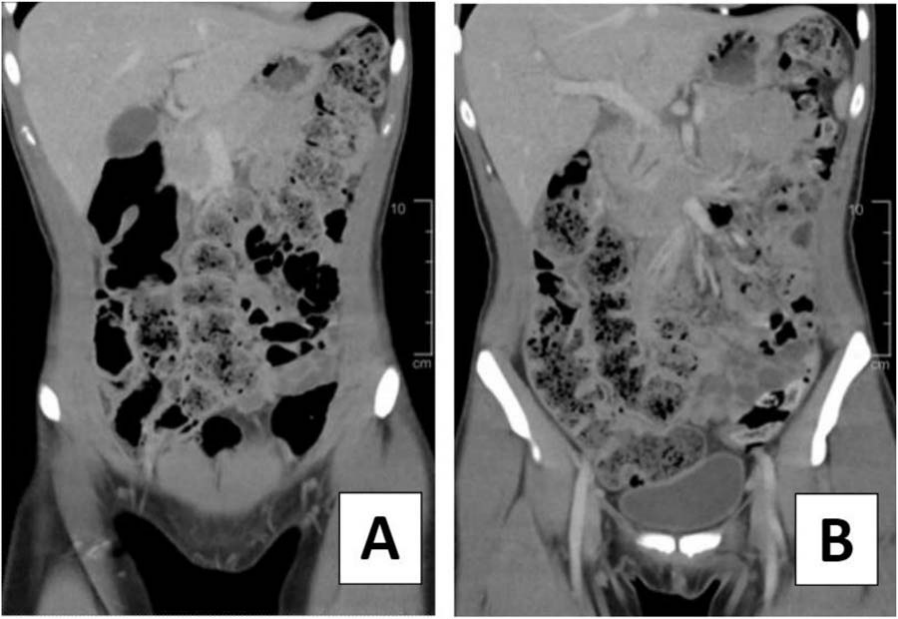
CT scan of 23-year-old female on hospital Day 1. (A, B) Coronal views depicting moderate to large fecal load.
